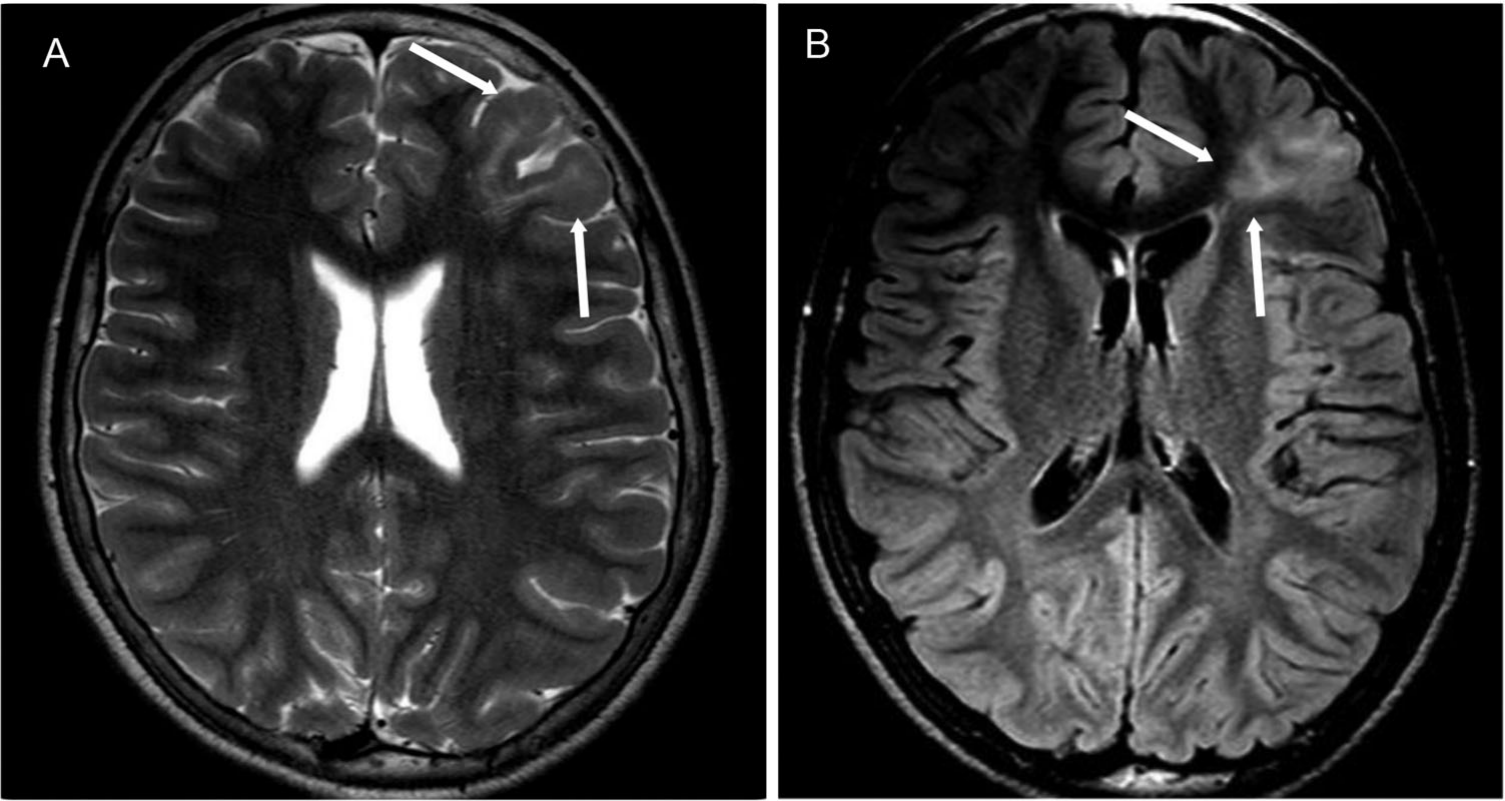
A typical MRI of focal transmantle cortical dysplasia in a 34-year-old woman. A) A coronal T2-weighted image demonstrating focal hyperintensity and the thickening of the right frontal cortex with a loss of demarcation between the gray and white matter (white arrow). B) A coronal T2 FLAIR showing a band of abnormal signal intensity extending from the cortex to the lateral ventricle (white arrows).
